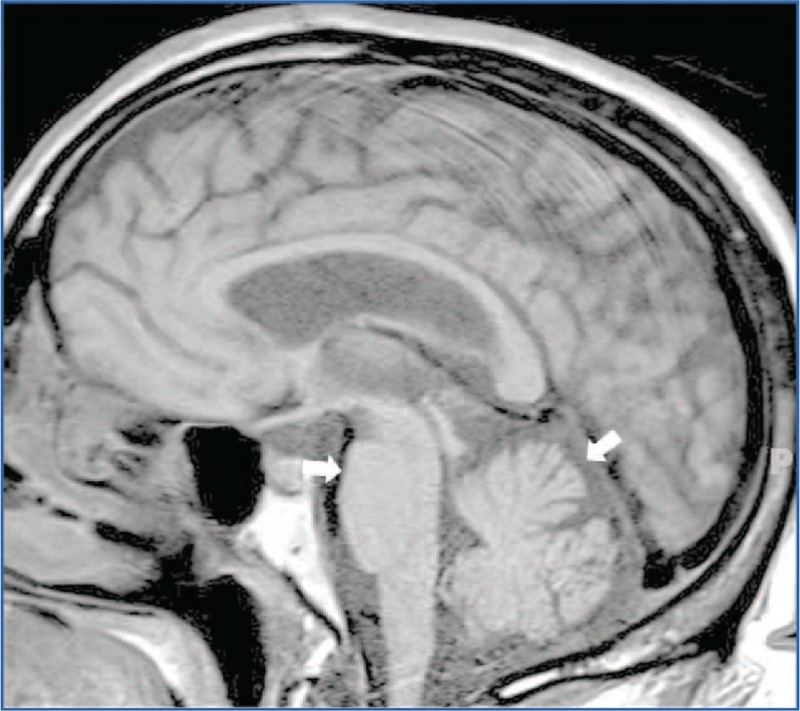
MRI findings in a patient with Multiple System Atrophy—Parkinsonian type. Sagittal view of the brain on MRI FLAIR sequence demonstrates that there is diffuse atrophy of the pons, cerebellum, and midbrain.
